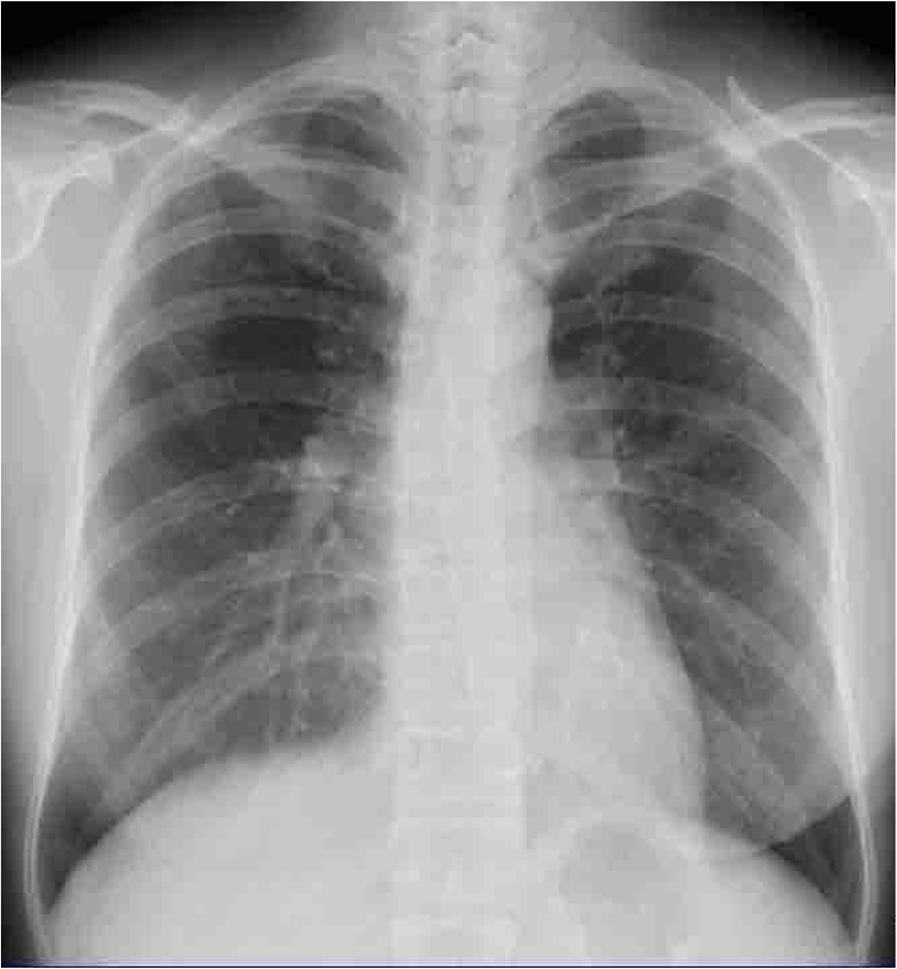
The chest X-ray shows slight peripheral infiltration shadows in both upper and middle lung fields.
